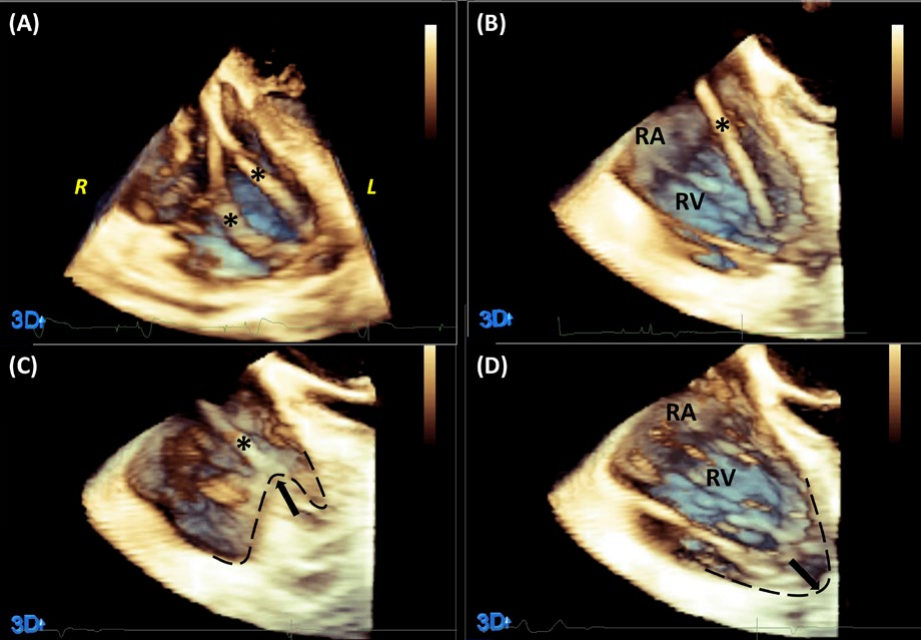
3D echocardiography focused on the right ventricle (RV) and right atrium during the ventricular lead extraction. A, Start of the procedure, with the two RV leads in situ (asterisk). B, The first lead was easily extracted; C, near inversion of the RV cavity (arrow, dashed contour) during pulling of the last lead; D, RV cavity re‐expanded after the lead detached (arrow, dashed contour)
